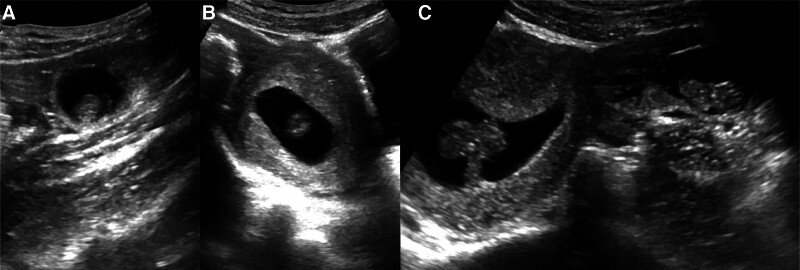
TAS results on day 66 of amenorrhea. (A) Showing HP. (B) Simultaneous showing HP on the same tangent plane. HP = heterotopic pregnancy, TAS = transabdominal sonography.
